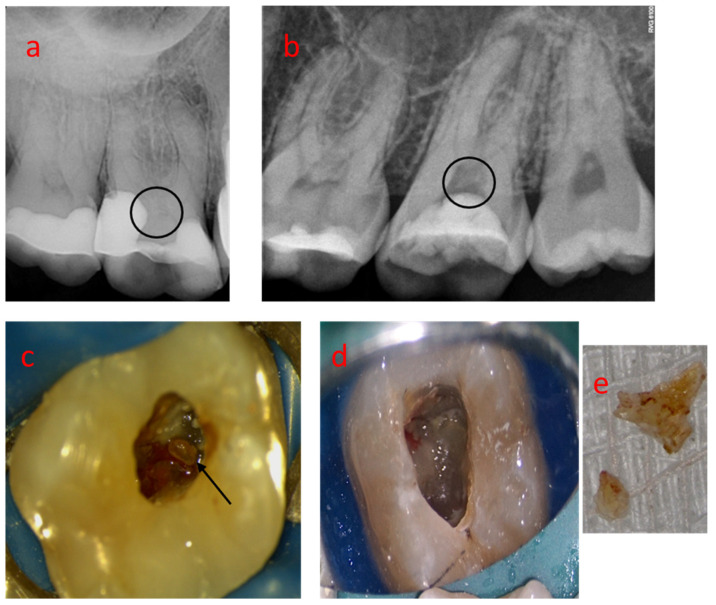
(a,b) Preoperative radiographs displaying dental pulp nodules within upper molars’ coronal pulp; (c) Pulp nodules visible during access (arrows); (d,e) A diffuse pulp nodule in a pulp cavity and after the removal.
